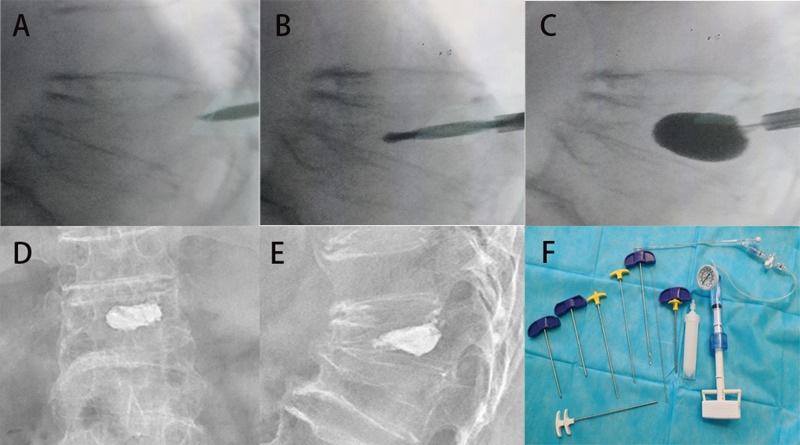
BTS surgical procedure for the treatment of a 75-year-old male patient with T12 vertebral body fracture. A, A puncture needle enters into the wedge T12 vertebra via the left pedicle. B, A bone drill was inserted in the fractured vertebral body to drill a circular hole as a working channel. C, An expandable cannula was inserted into the fractured vertebral body, and the balloon was slowly inflated. D and E, Posterior-anterior and lateral X-ray films after the BTS surgical procedure. F, The BTS instruments.
